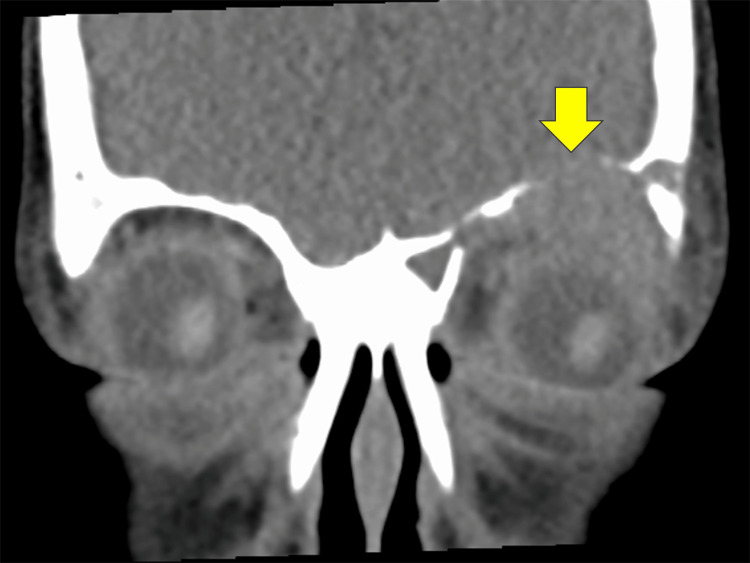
A coronal computed tomography image The image shows soft tissue mass in the superolateral orbit with an osteolytic lesion (arrow).
